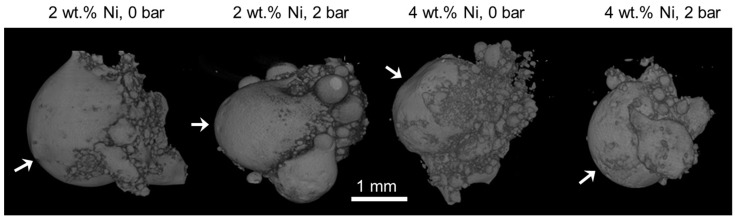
Overall shape of the produced samples reconstructured from μ-CT scans. The arrows indicate the direction of laser beam incidence during the fabrication process.
